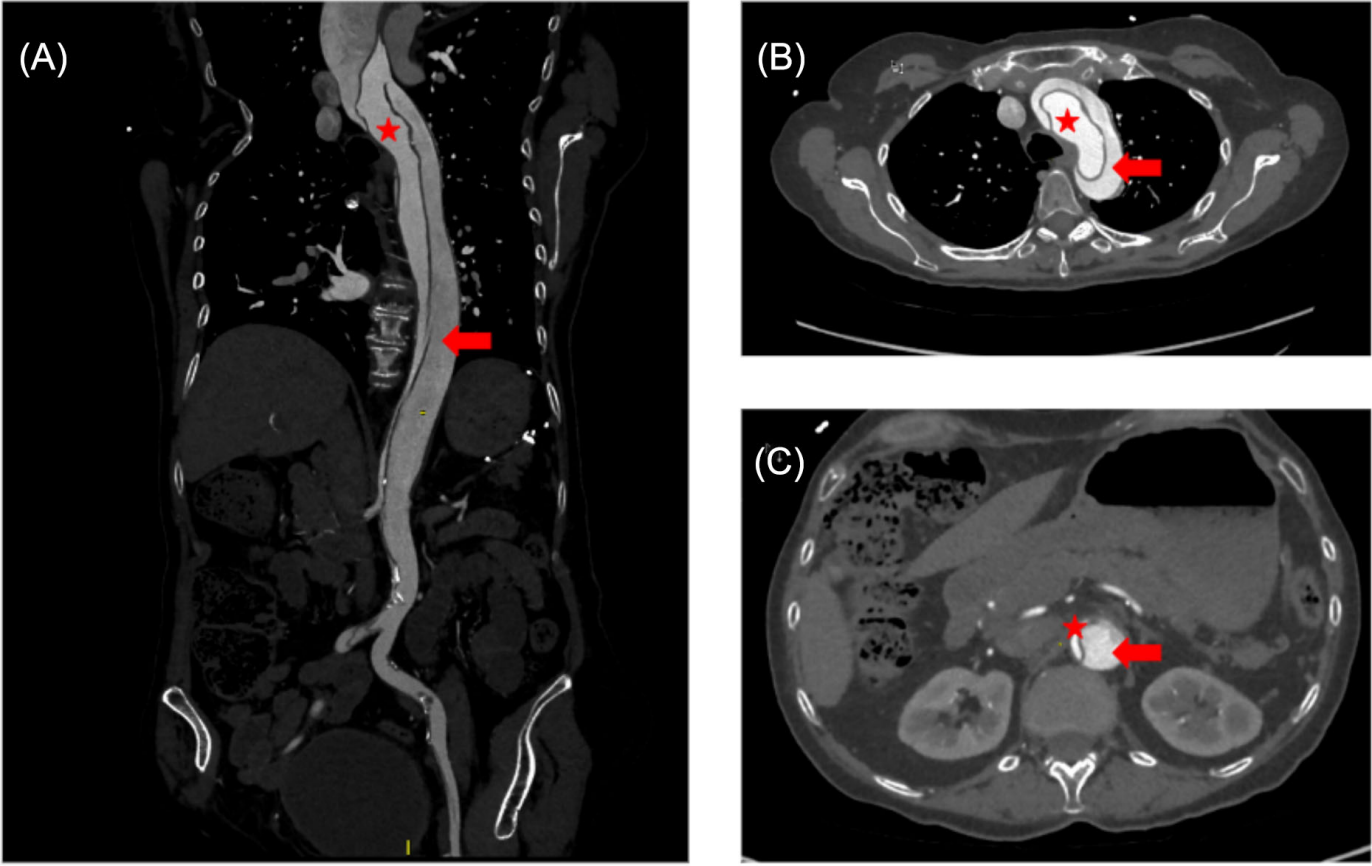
Computed tomography imaging of acute aortic dissection. (A) curvilinear reconstruction of the descending aorta showing AAD involving thoracic aorta, abdominal aorta and iliac artery, (B) thoracic aorta, (C) abdominal aorta. Red stars represent true lumen and red arrows represent false lumen.
